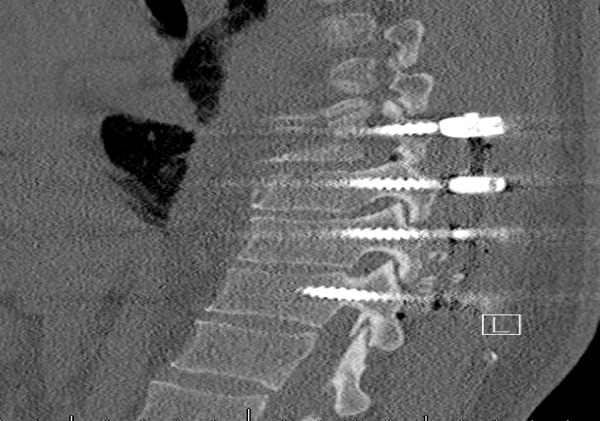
Parasagittal CT demonstrating the position of thoracic screws with proposed entry point and sagittal trajectory.
